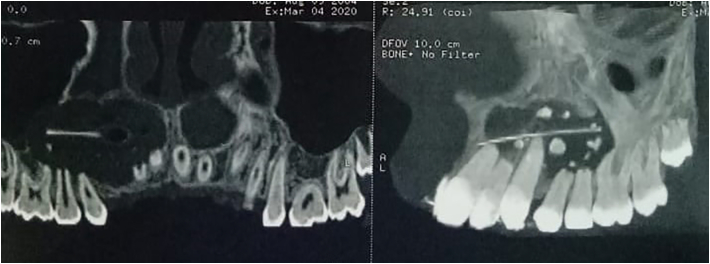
CT scan showing an image of a lytic lesion pushing back the floor of the right maxillary sinus with the presence of hyperdensities in its center.
